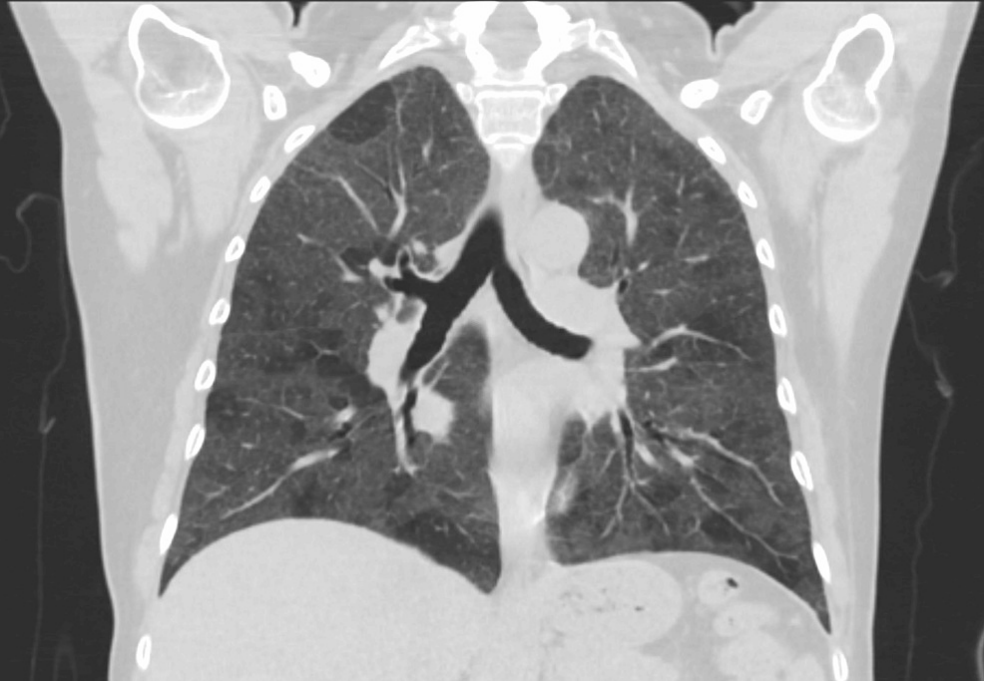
CT chest showing hazy ground glass opacity bilaterally
